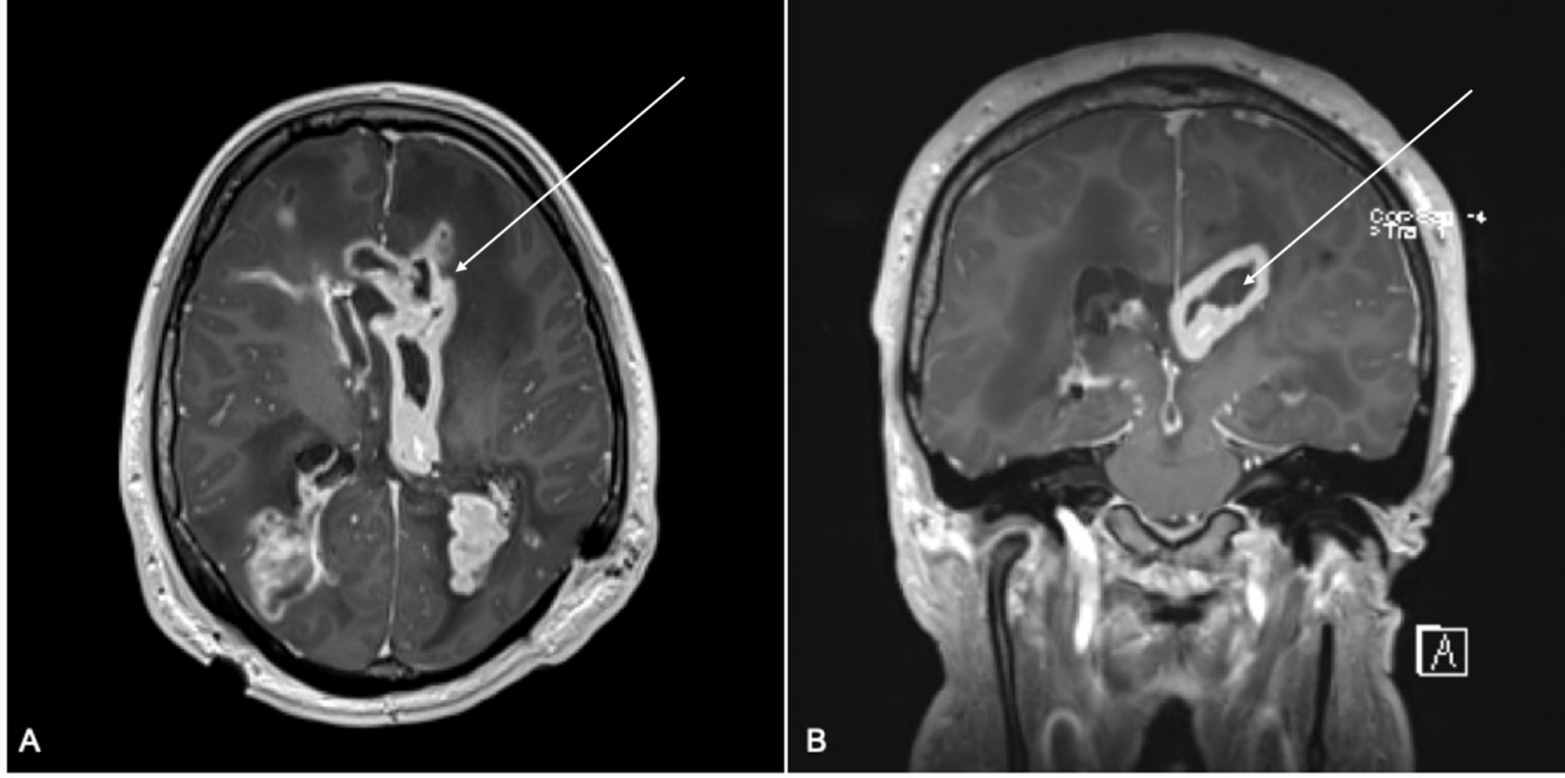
MRI T1 with contrast MRI T1 with contrast showing severe ventriculitis (arrow) and adjacent cerebral inflammatory changes with edema in axial (A) and coronal (B) sequences.
